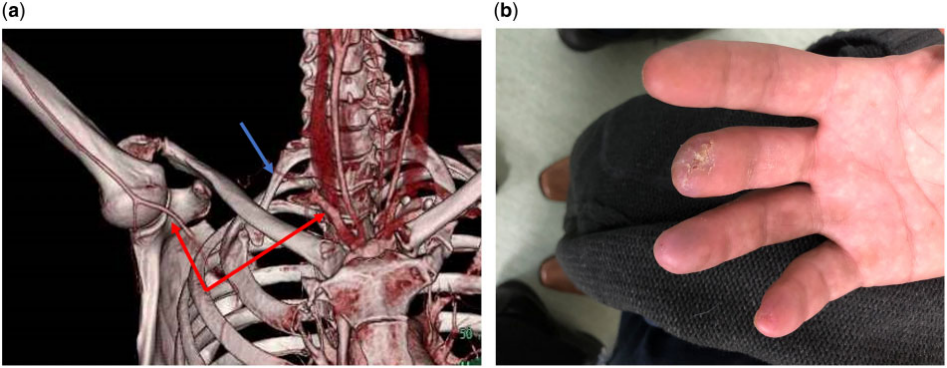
Radiological and clinical findings of Case 2 (A) CT angiogram of the right upper limb showing unilateral right cervical rib (blue arrow) and compression of the right subclavian artery in the abduction position (red arrows). (B) Right hand 6 months postoperatively, with healed terminalization of the middle finger.
